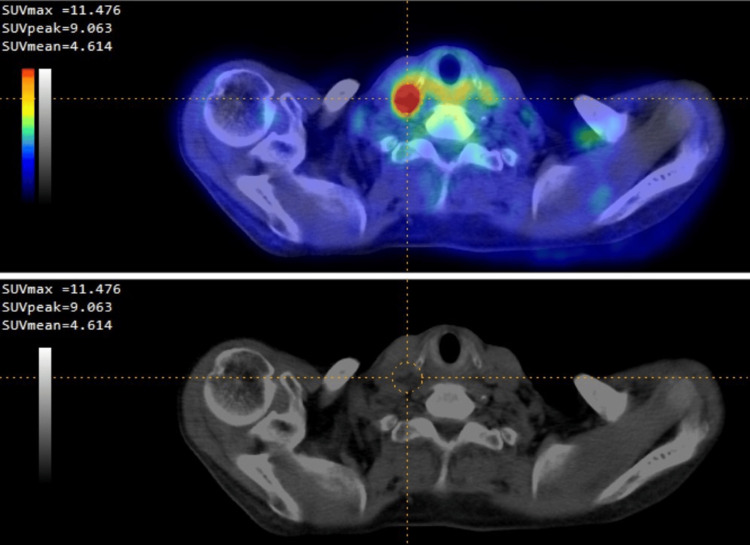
Quantitative analysis of FDG PET imaging in a patient with superficial esophageal squamous cell carcinoma using Metavol software The upper panel displays a Maximum Intensity Projection (MIP) image of FDG uptake. A red cross mark highlights areas of high FDG activity associated with the tumor. The lower panel shows the corresponding CT image with a red cross mark indicating the tumor's location. FDG: 18F-fluorodeoxyglucose; PET: positron emission tomography
